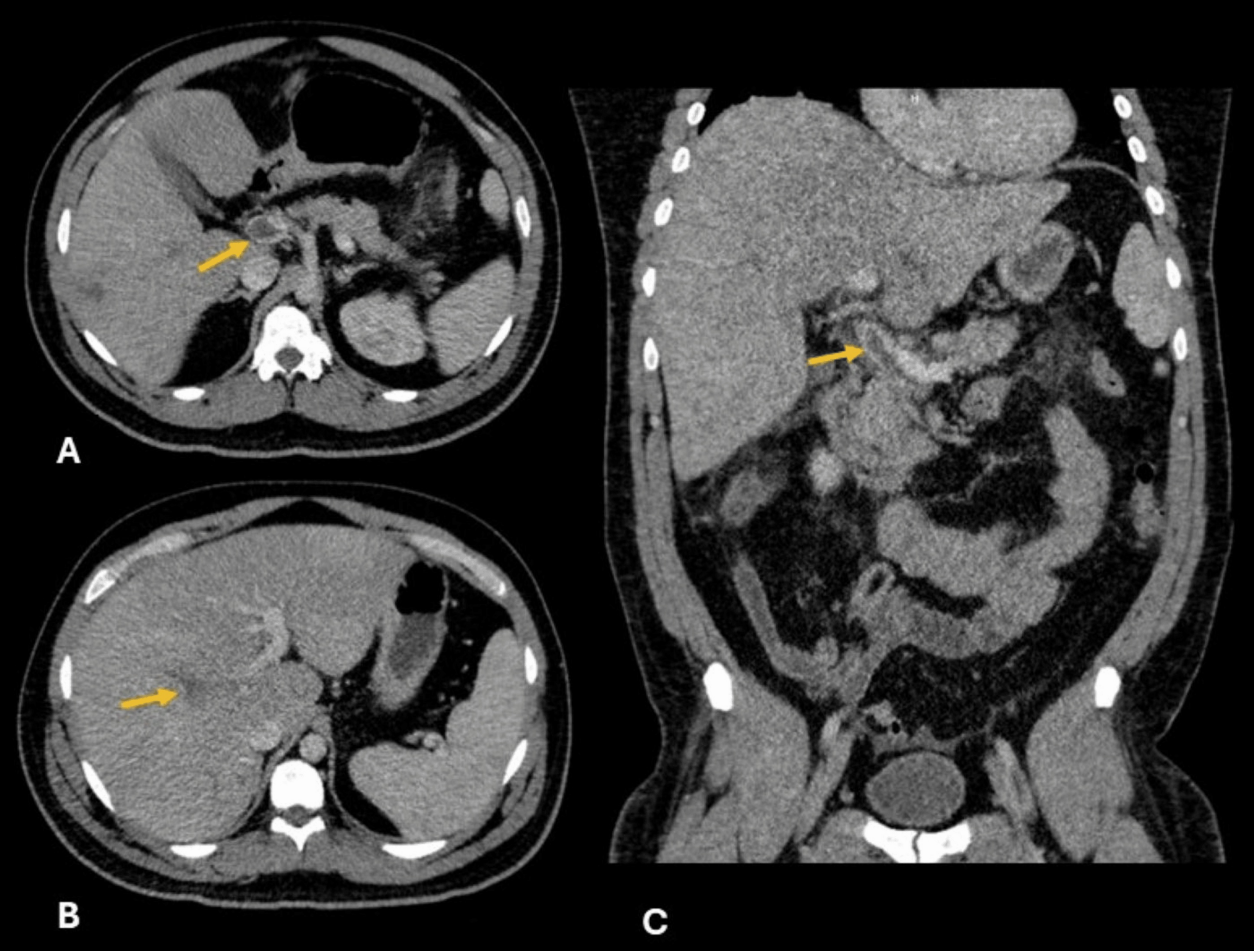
Axial and coronal, portal-phase contrast-enhanced abdominal CT Central filling defects in the main portal vein (A, yellow arrow), right portal branches (B, yellow arrow), and at the splenomesenteric junction and superior mesenteric vein (C, yellow arrow).
